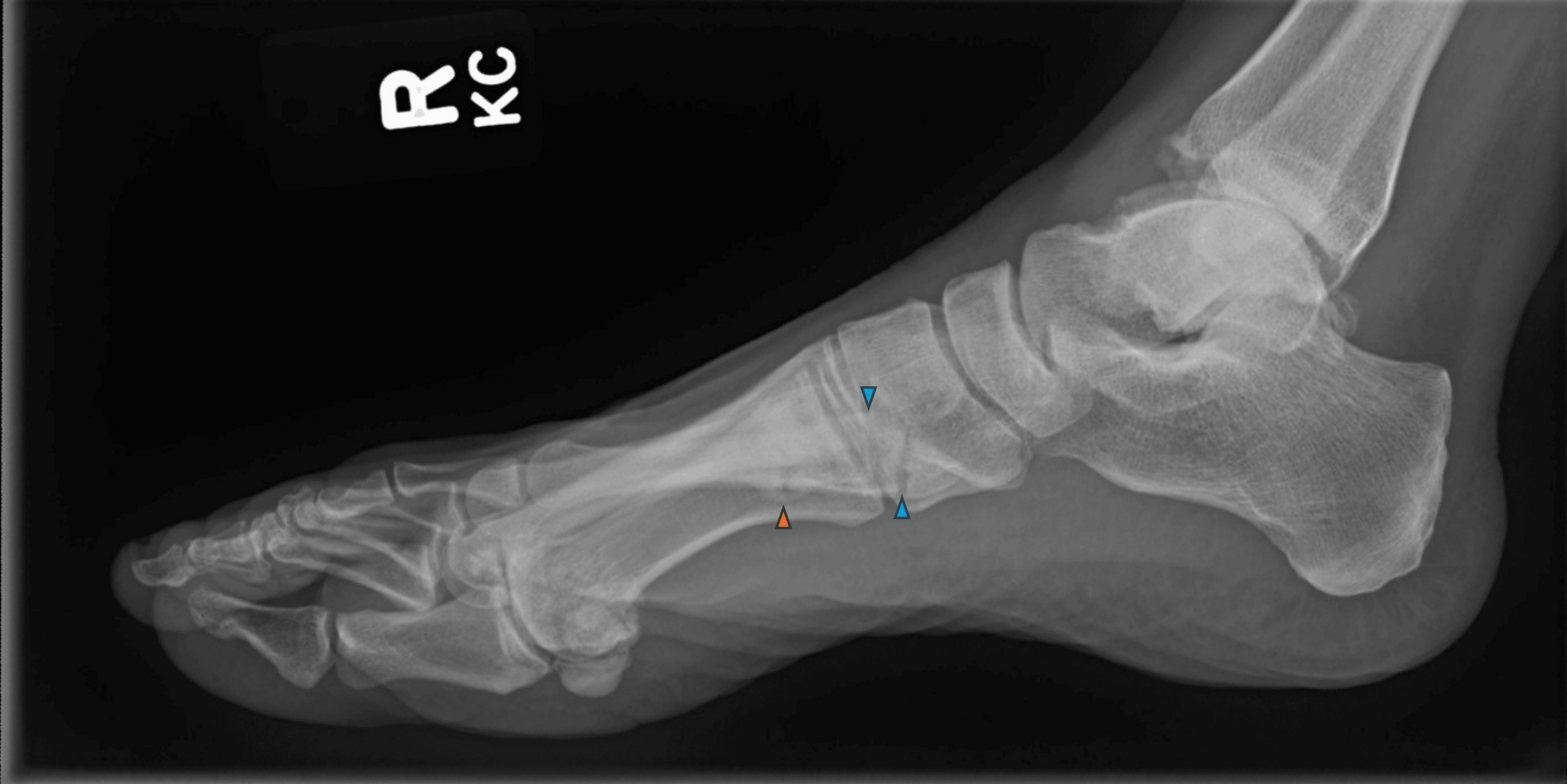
Radiograph demonstrating normal alignment and mineralization of metatarsals in case 1. There is a non-displaced fracture of the base of the fifth metatarsal bone with two fracture lines visible (blue arrowheads), and no definite intra-articular extension. An old non-displaced healed fracture of the proximal meta-diaphysis of the fourth metatarsal bone with sclerosis at the fracture margin and callus formation are also visualized (orange arrowhead).
